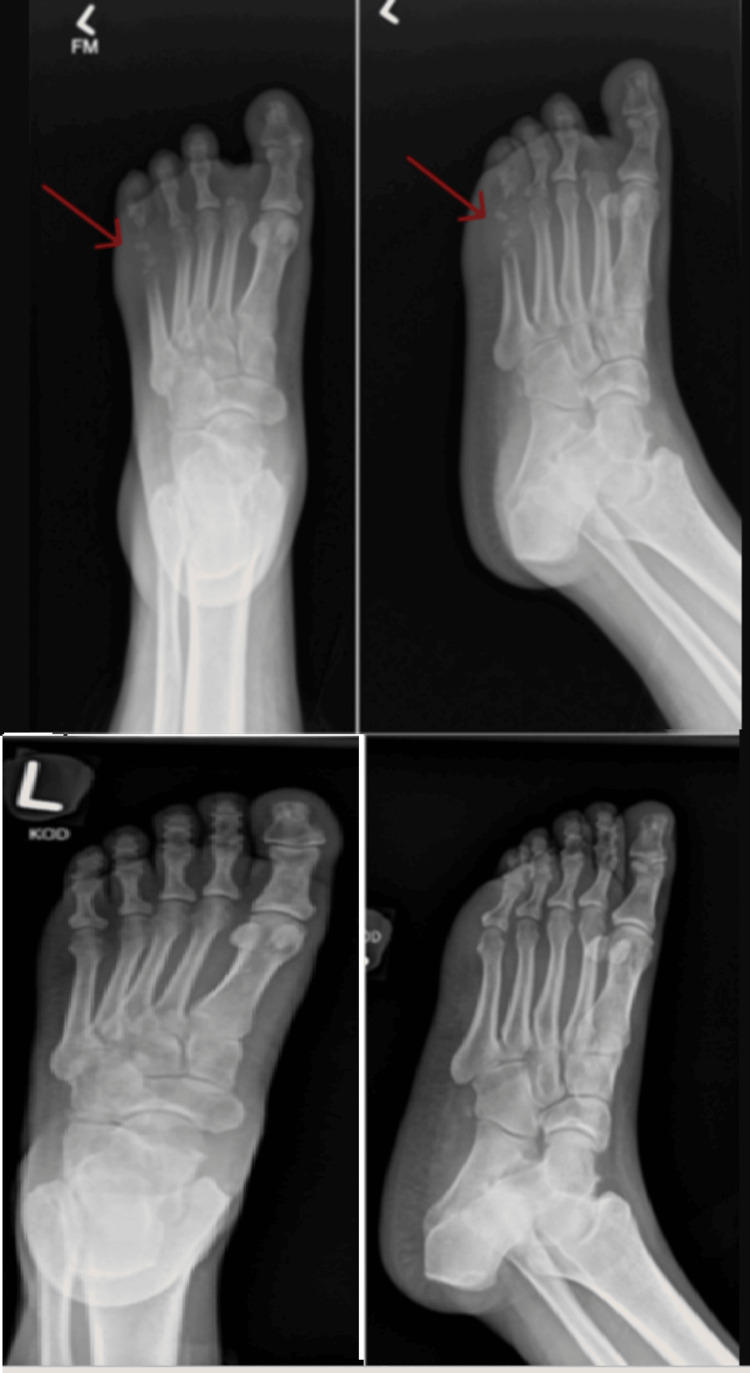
Radiographs of the left foot, demonstrating osteolysis of the fifth distal metatarsal with probable extension to the distal phalanx concerning for acute osteomyelitis (top row, arrow). Radiographs of the left foot for comparison from nine years before (bottom row)
